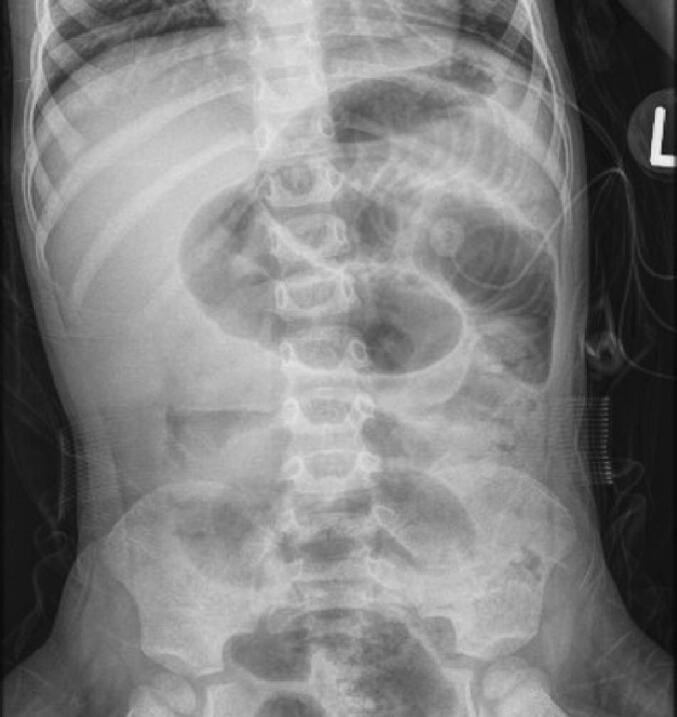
Abdominal radiograph showing dilated loops of bowel concerning for obstruction.
